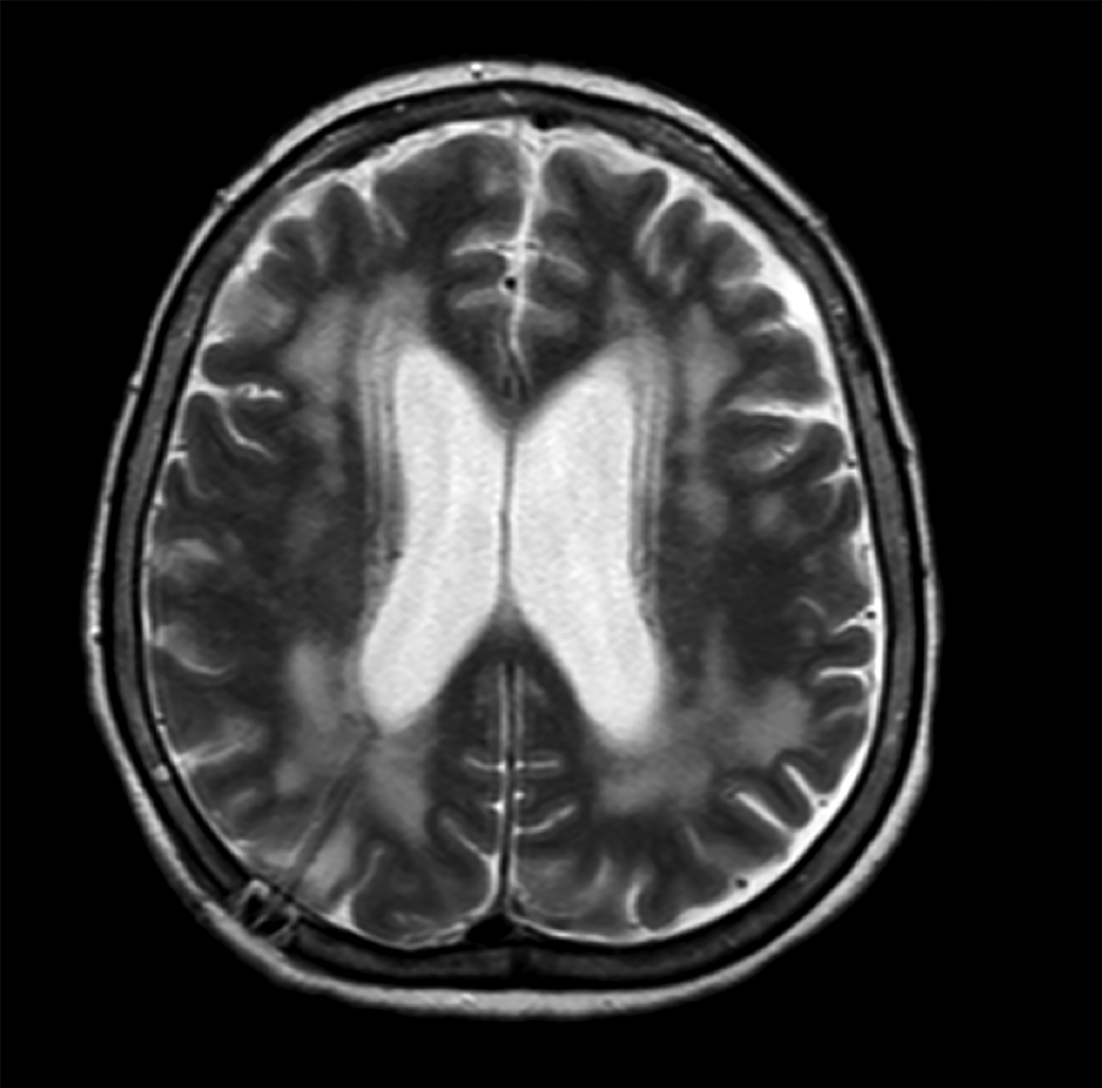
Magnetic resonance imaging revealing quadriventricular hydrocephalus, non‐specific periventricular change, and diffuse meningeal enhancement.
